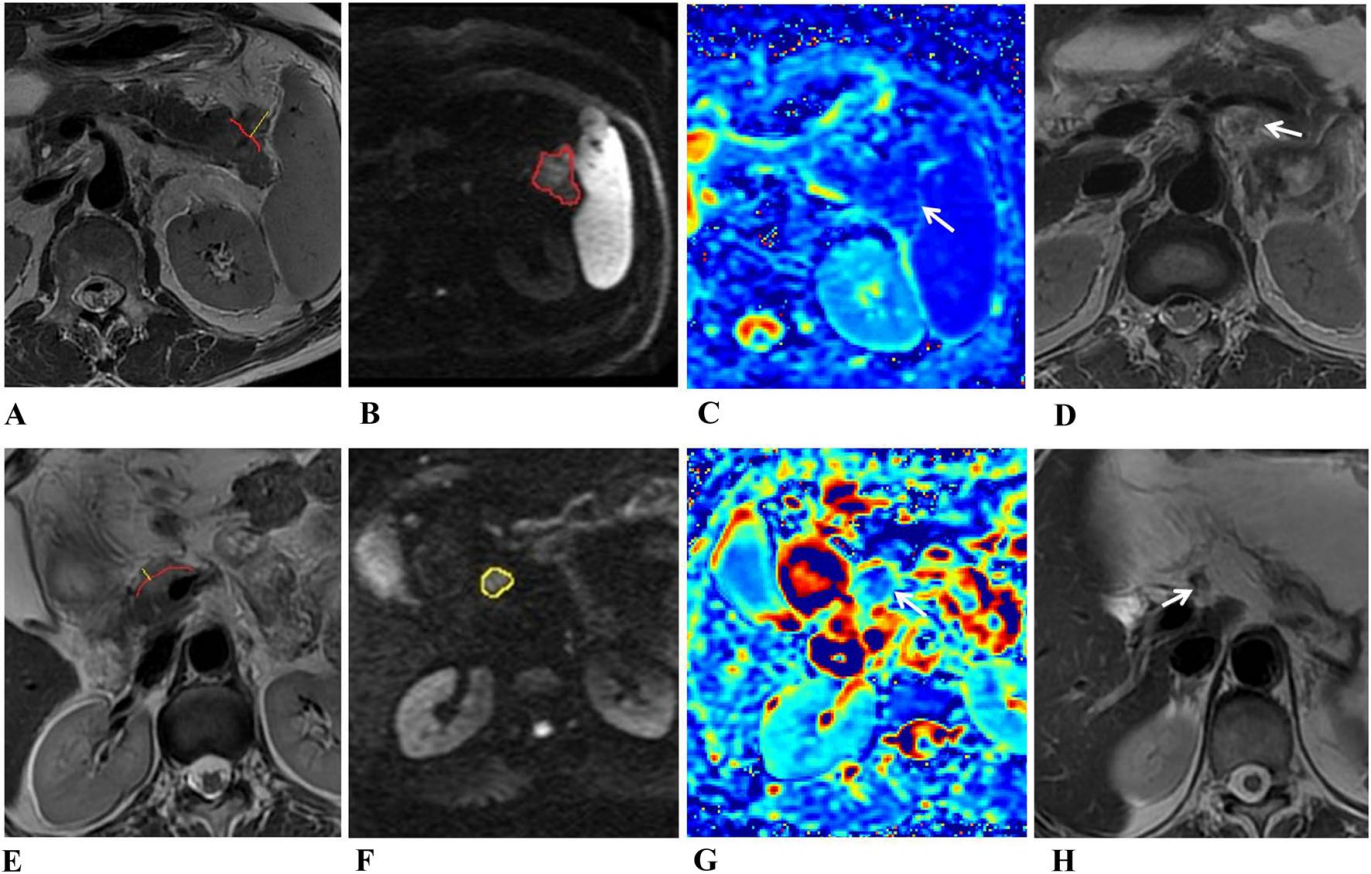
(a–d) MR images of a 58-year-old man with pancreatic carcinoma with positive LNM. a Axial T2-weighted image showed an irregular high signal intensity tumor in the tail of the pancreas; the extrapancreatic distance (yellow line) of tumor invasion was 17 mm. b DWI with b = 1500 s/mm^2^ showed a hyperintense tumor; the ROI was drawn, including the entire tumor. c On the MD parametric map, the tumor showed an isointense signal with a value of 1.54 × 10^–3^ mm^2^/s, which was less than the cutoff value of 1.74 × 10^–3^ mm^2^/s. d On axial T2-weighted image, diameter of LN around the splenic artery was 7 mm (arrow). e–h MR images in a 56-year-old woman of pancreatic carcinoma with negative LNM. e Axial T2-weighted image showed a well-defined high signal intensity tumor in the head of the pancreas; the extrapancreatic distance (yellow line) of tumor invasion was 6 mm. f DWI with b = 1500 s/mm^2^ showed a hyperintense tumor; the ROI was drawn, including the entire tumor. g On the MD parametric map, the tumor showed an isointense signal with a value of 2.70 × 10^–3^ mm^2^/s. h Axial T2-weighted image showed LN with a diameter of 5 mm around the pancreatic head
